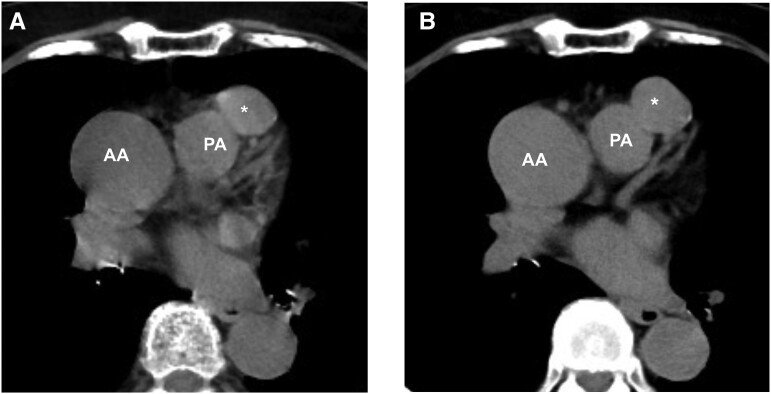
Preoperative computed tomography 2 years prior (*A*) and at referral to our hospital (*B*). The asterisk indicates a large aneurysm. AA, ascending aorta; PA, main pulmonary artery.
